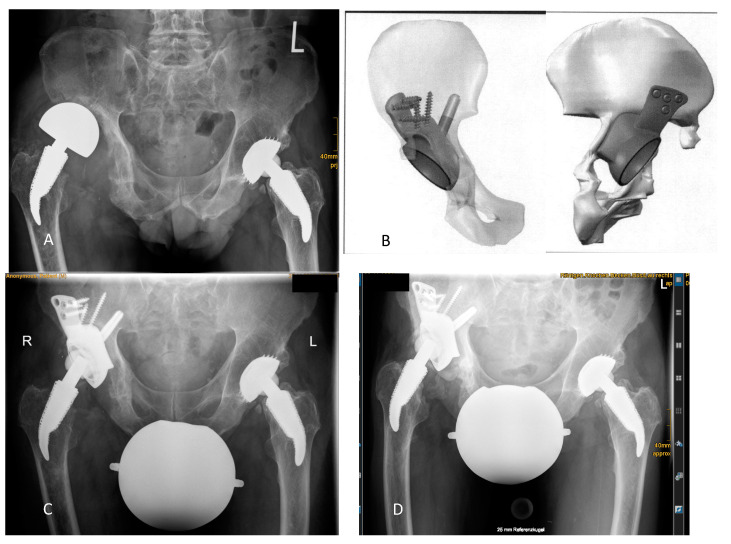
Radiolucency lines without need for revision: (A) Preoperative radiographic situation showed the acetabular “up-and-out” defect after implantation of a large head because of acetabular bone loss. (B) Anterior to posterior (left) and posterior to anterior (right) views show the intended proximalization of the COR in the virtual 3D reconstruction. The cup is not placed at the level of the Kohler’s tear drop. (C) Radiograph after revision and CMAC implantation showed restoration of leg length with a high COR. (D) Significant radiolucency lines developed around the whole implant at 3 years of follow-up. Although implant migration cannot be excluded, the patient was not revised because he reported daily walks of up to 10 km supported by a cane. Thus, this case was not considered a failure.
